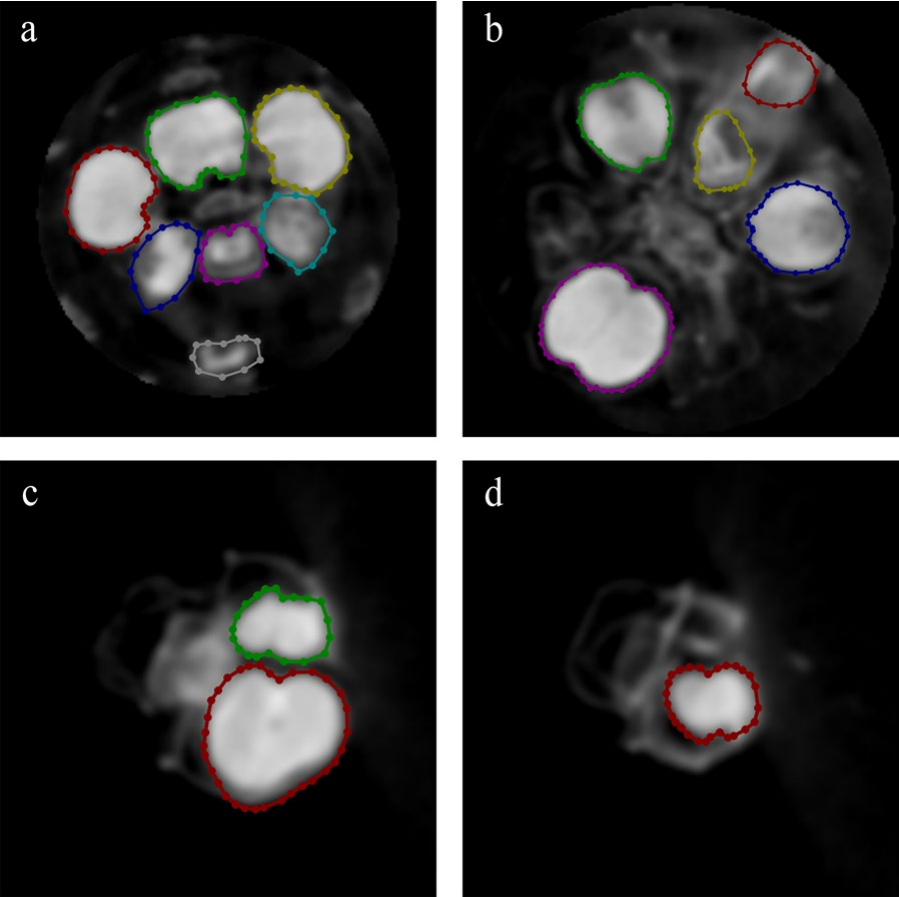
Samples of images of manually labeled regions of grains from a CT slice. a, d Labeled slice image of barley variety s17350. b Labeled slice image of wheat cultivar s113095. c Labeled slice image of wheat cultivar D3
